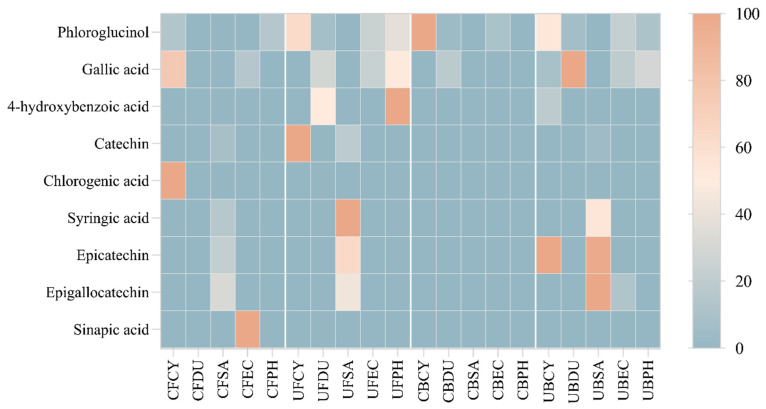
Heat map distribution of free and bound phenolic extract of brown seaweed samples using conventional and ultrasonication extraction methods. CF, Conventional Free; UF, Ultrasonication Free; CB, Conventional Bound; UB, Ultrasonication-Bound; CY, *Cytospora* sp.; DU, *Durvillaea potatorum*; SA, *Sargassum fallax*; EC, *Ecklonia radiata*; PH, *Phyllospora comosa*. Results are expressed in mg/mL.
